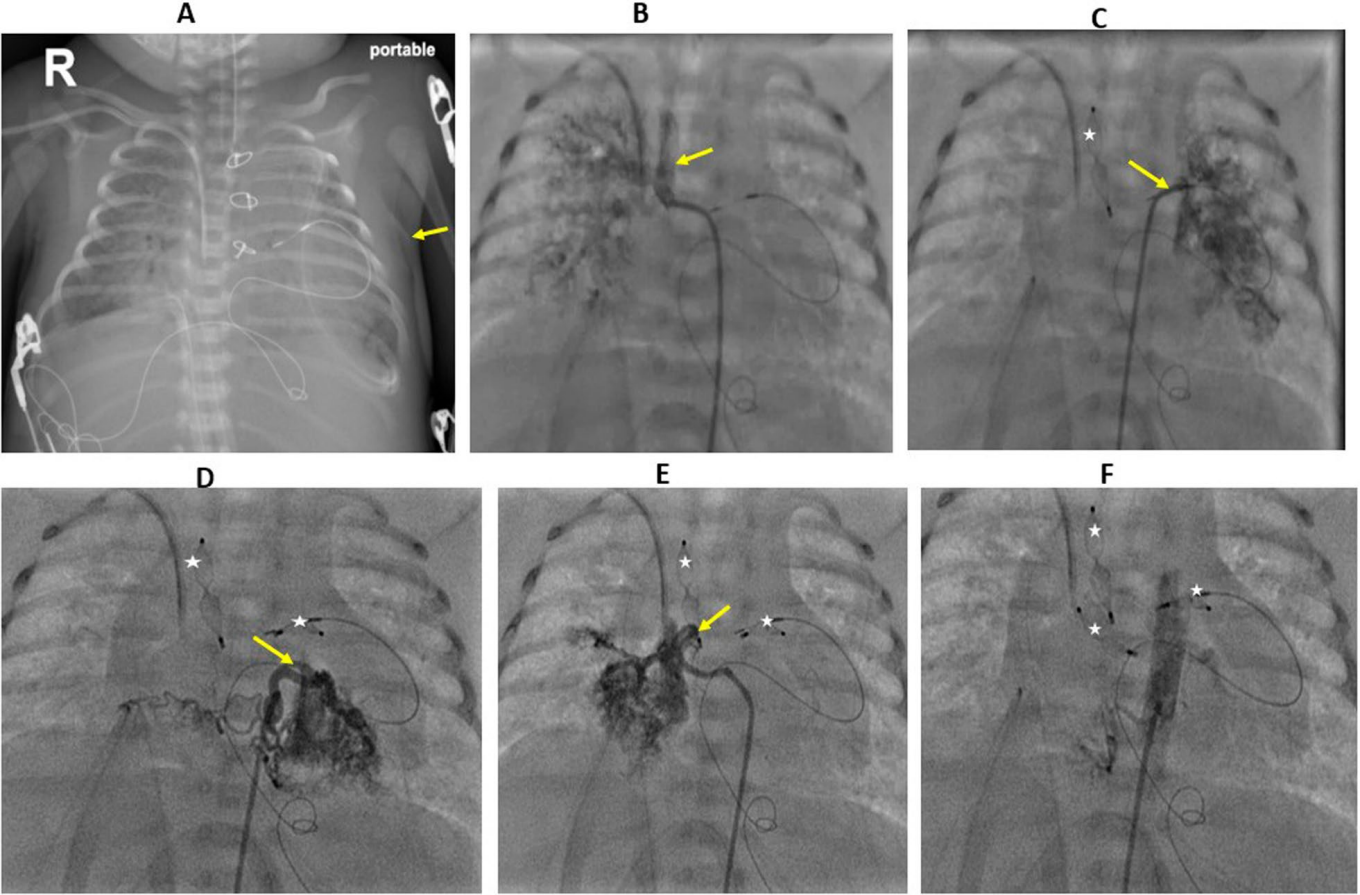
Roentgenogram and angiography before and after the closure of MAPCAs: A chest X-ray after ASO showed pulmonary edema with significant skin edema (yellow arrow). B selective angiography showing a large MAPCA supplying the right upper lung lobe (yellow arrow). C selective angiography showing a large MAPCA supplying the left upper lung lobe (yellow arrow) with vascular plug seen closing the MAPCAs of right upper lobe (white asterisk). D selective angiography showing MAPCA supplying the left lower lung zone with vascular plugs seen occluding MAPCAS for upper left and right lungs (white asterisks). E selective angiography showing large MAPCA supplying the right lower lung lobe (yellow arrow). F Successful closure of 3 MAPCAs using three vascular plugs (white asterisks). ASO: arterial switch operation, MAPCAs: Major aortopulmonary collateral arteries
